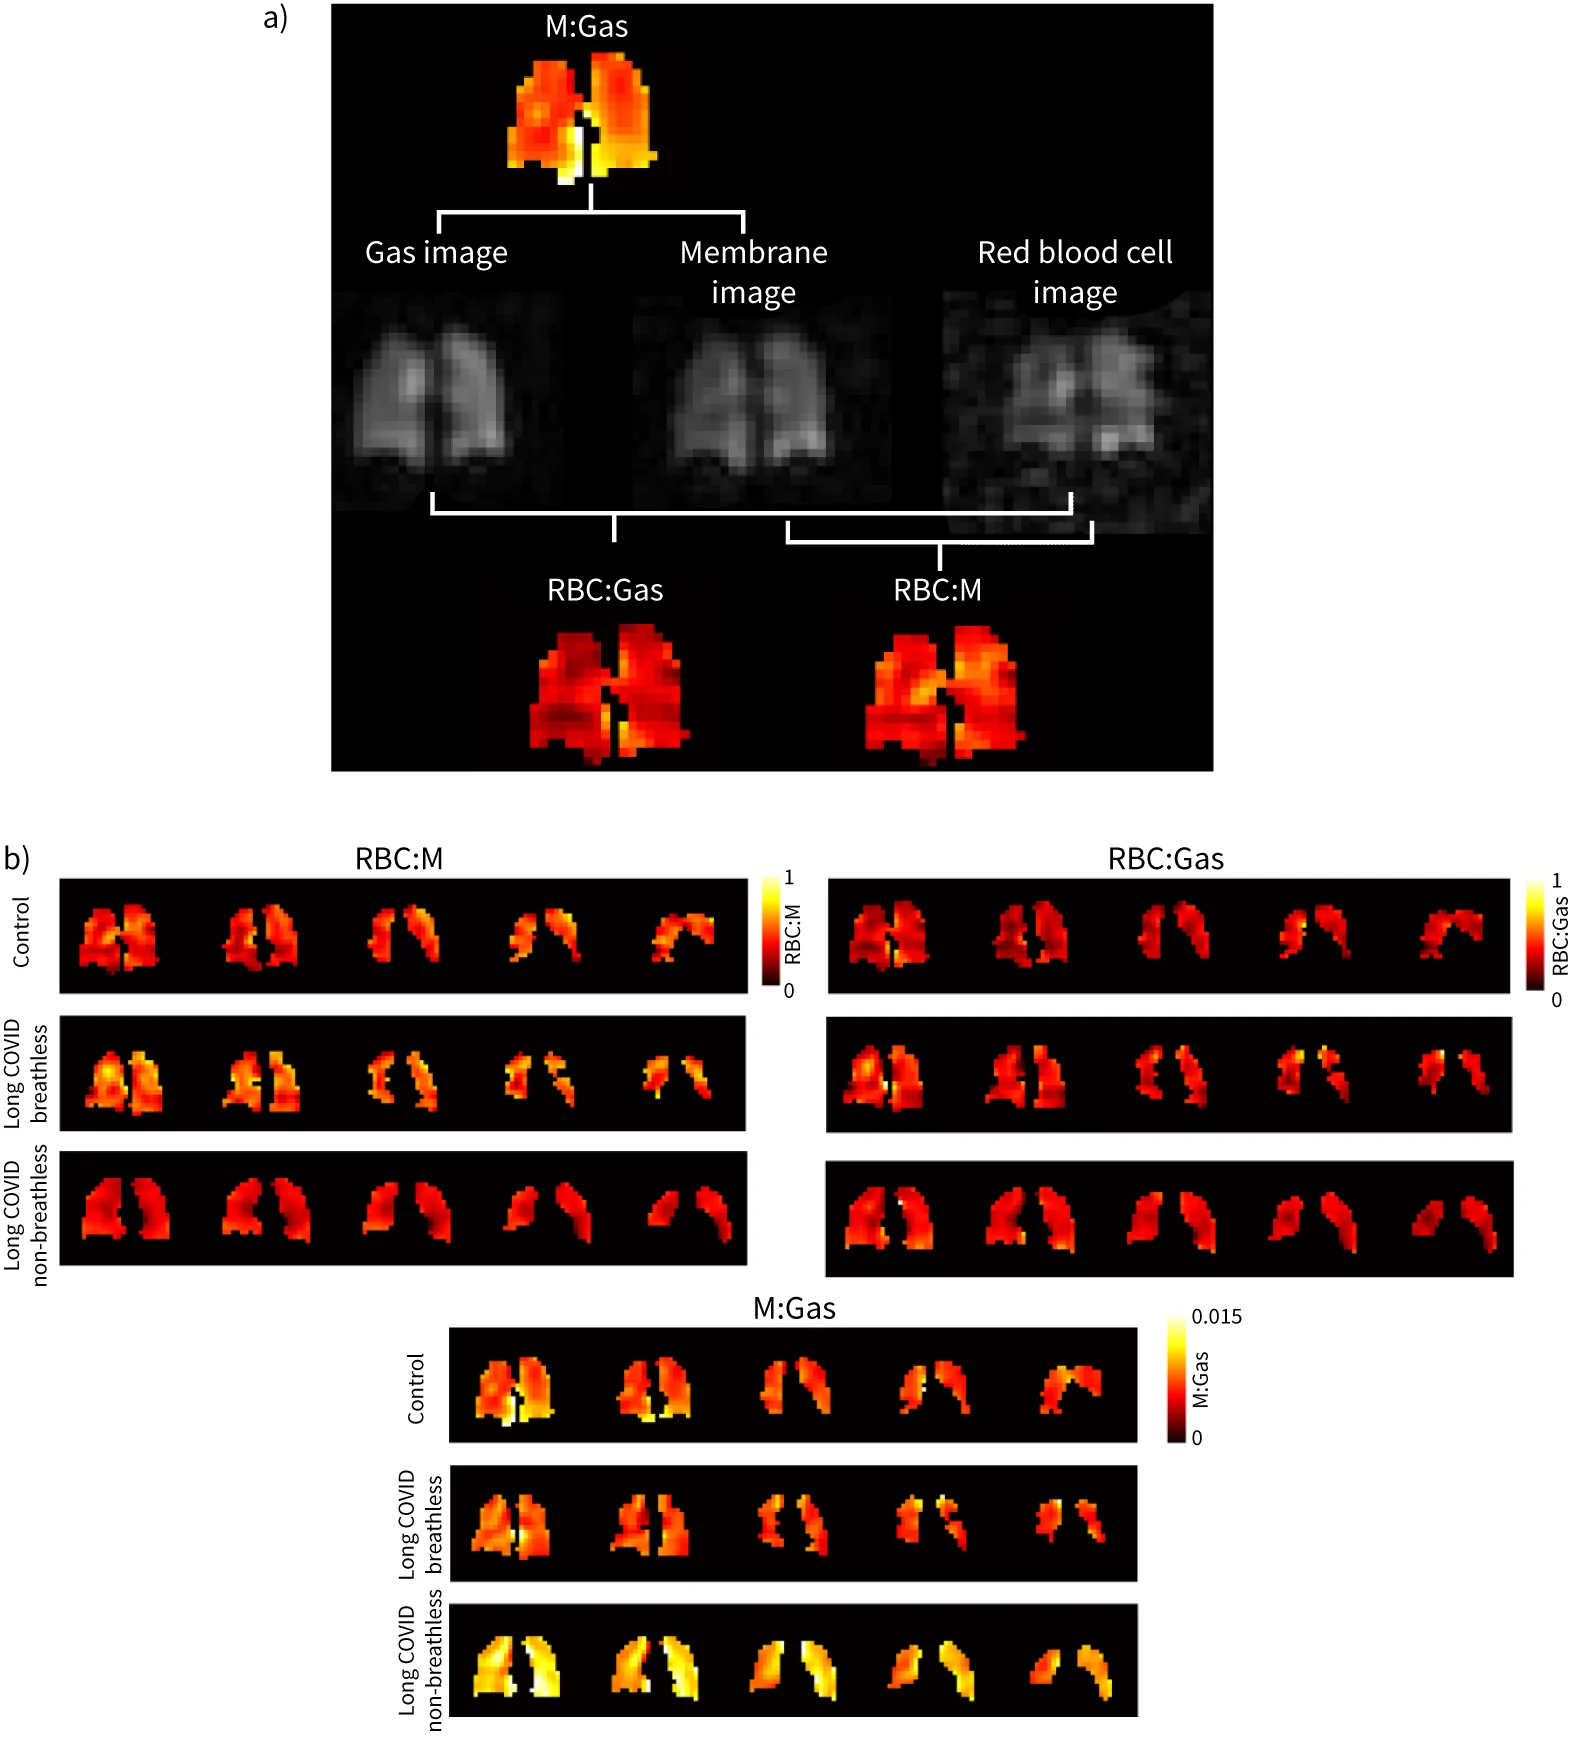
Hyperpolarised xenon pulmonary MRI (^129^Xe-MRI) image maps. a) Figure to demonstrate gas, membrane and red blood cell (RBC) ^129^Xe-MRI image maps and how the ratios of RBC:Gas, RBC:M and M:Gas are calculated from gas and RBC measurements and membrane and RBC uptake, accordingly. b) Representative image maps of the RBC:M, RBC:Gas and M:Gas mean ratio maps of a control (top), breathless long COVID (middle) and non-breathless long COVID (bottom) participant.
